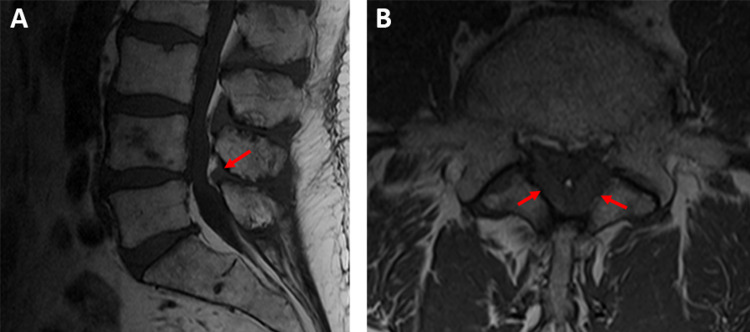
T1-weighted magnetic resonance images (A) Mid-sagittal view depicting severe L4-5 stenosis and ossification of the ligamentum flavum (red arrows). (B) Transverse view depicting severe L4-5 stenosis and ossification of the ligamentum flavum (red arrows).
